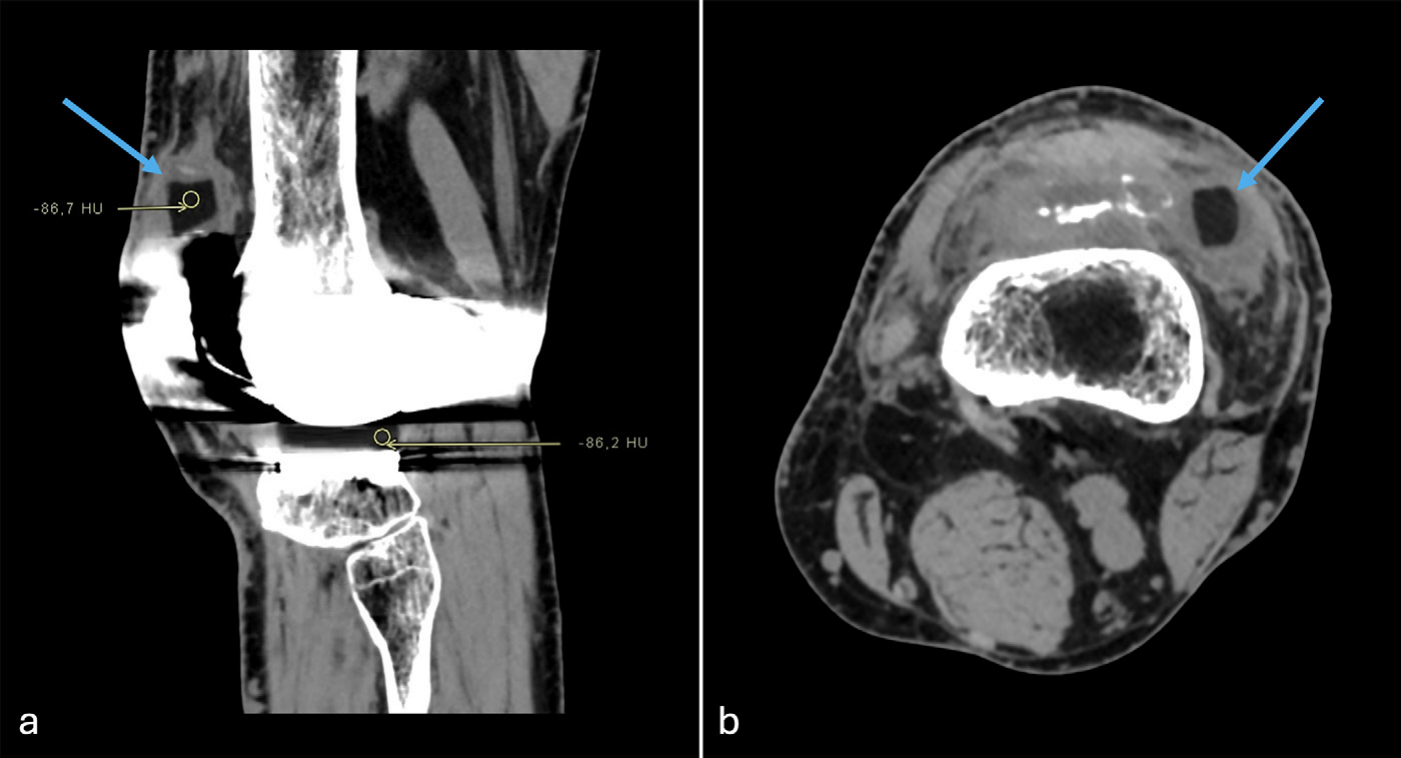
CT images displayed in a soft-tissue window demonstrating a displaced polyethylene tibial post fragment (blue arrows). (a) Sagittal image showing region-of-interest measurements within the displaced fragment and the intact tibial insert, revealing similar low attenuation values (approximately −86 HU), consistent with polyethylene. (b) Axial image demonstrating the well-defined displaced fragment in the lateral suprapatellar region.
